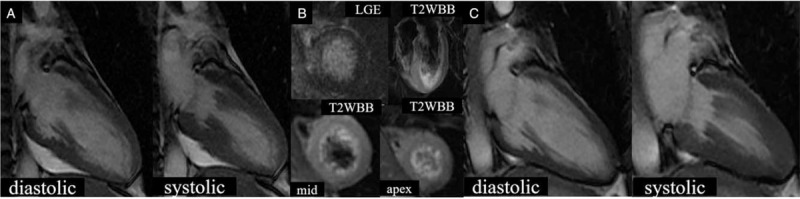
(A) Apical ballooning observed on cardiovascular magnetic resonance imaging (CMR) on the fourth day of hospitalization. (B) Late gadolinium enhancement and T2-weighted black blood are observed on CMR on day 4 of hospitalization. (C) Second CMR 49 days after the first CMR.
